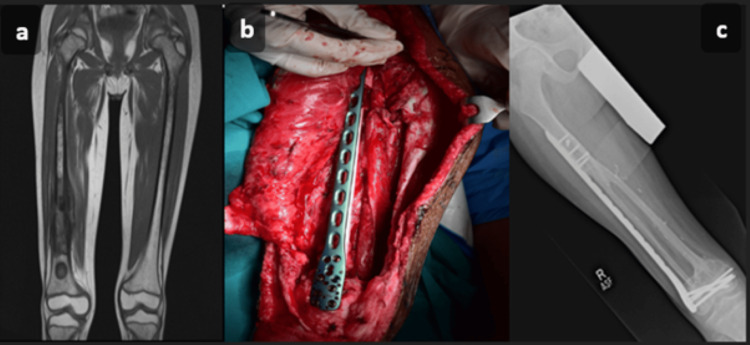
(a) Eleven-year-old with right femur osteosarcoma seen on magnetic resonance imaging (pre-operative). (b) Right femur bone defect after insetting of free fibula flap (intra-operative). (c) Plain radiograph with the fibula set in place (immediate post-operative) (Photography and Designed by: Safdar Ali Sheikh).
